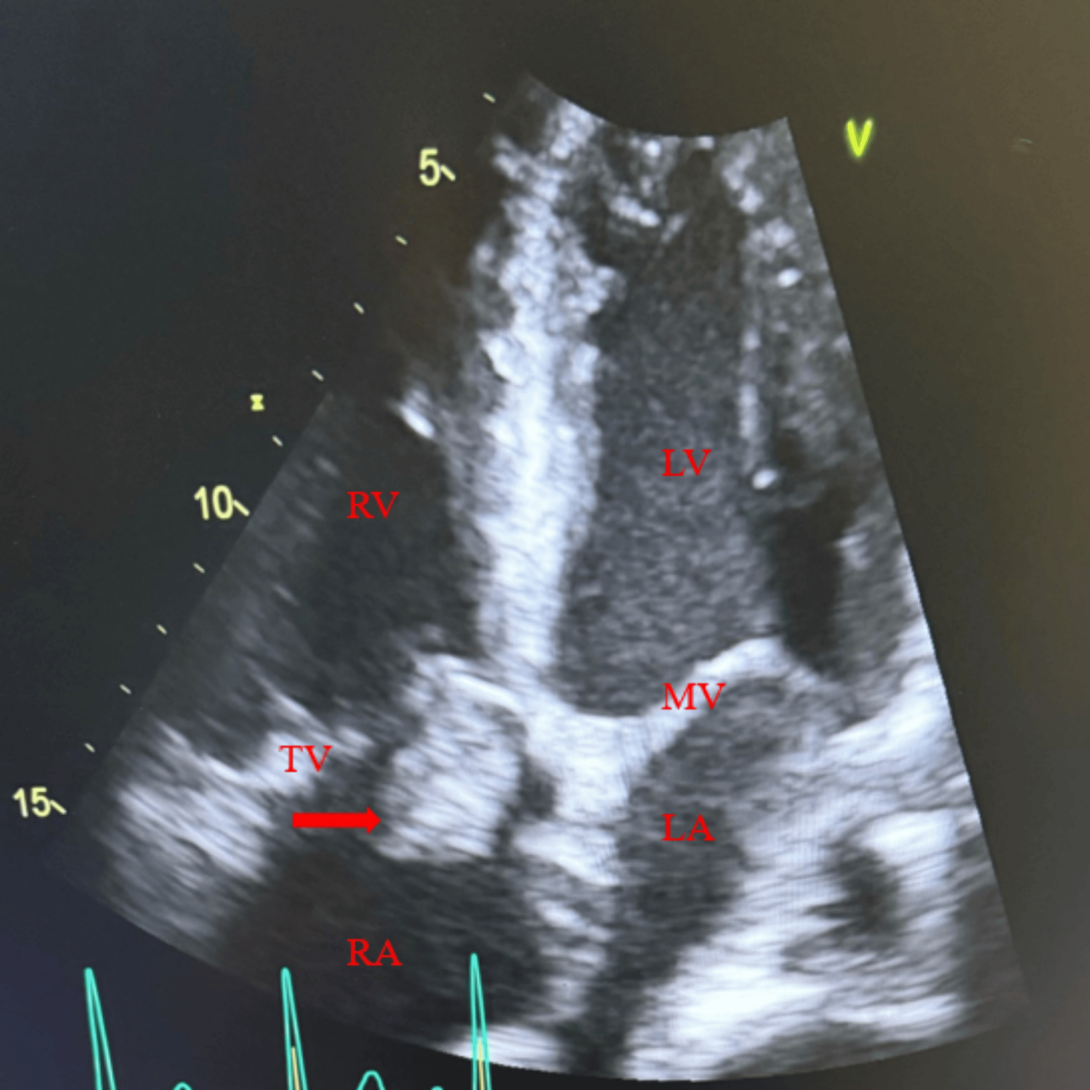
Transthoracic echocardiography, apical four chamber view showing tricuspid vegetation. Red arrow pointing to the tricuspid valve vegetation. RV, right ventricular; LV, left ventricular; TV, tricuspid valve; MV, mitral valve; RA, right atrium; LA, left atrium.
